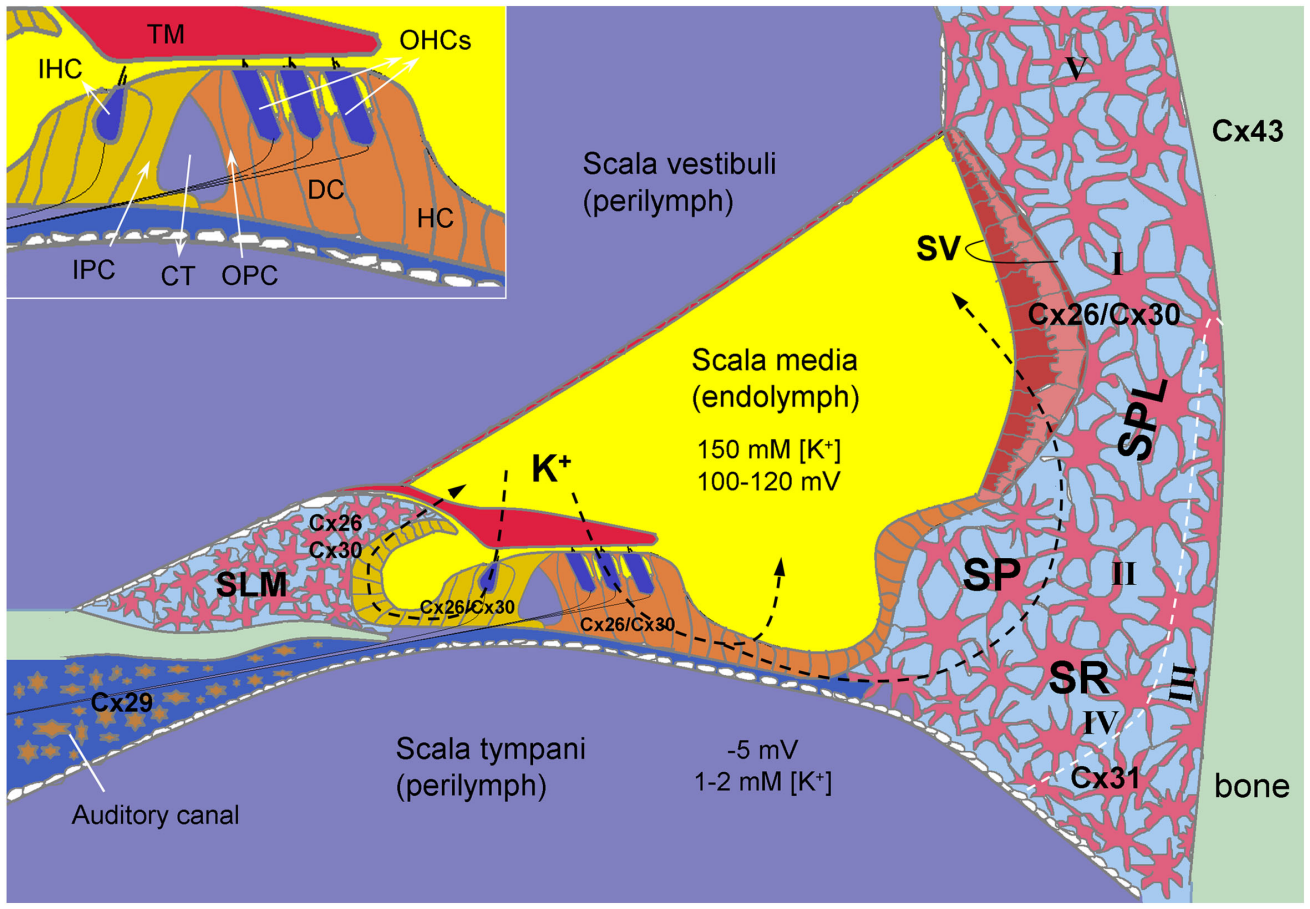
Connexin expression and hypothesized K^+^-recycling in the cochlea. Cx26 and Cx30 co-localized in supporting cells of the organ of Corti, the spiral limbus (SLM), the stria vascularis (SV), and fibrocytes of the spiral ligament (SPL). Cx31 is localized at type II and IV fibrocytes in the subcentral region (SR) below the spiral prominence (SP). Cx43 is expressed in the bone of the otic capsule. Cx29 is localized only at the Schwann cells wrapping the spiral ganglion neurons in the auditory canal. However, hair cells have no connexin expression. Inset: the organ of Corti. CT, cochlear tunnel; DC, Deiters cell; HC, Hensen cell; IHC, inner hair cell; IPC, inner pillar cell; OHCs, outer hair cells; OPC, outer pillar cell; TM, tectorial membrane; I–V, type I–V fibrocytes. Modified from Forge et al. (2003), Cohen-Salmon et al. (2004), Zhao and Yu (2006), and Liu and Zhao (2008).
